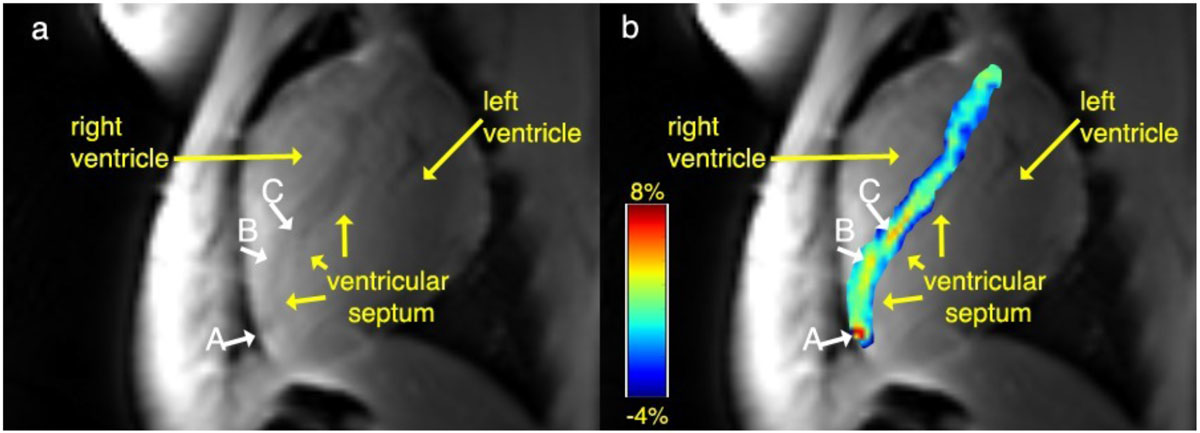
a| An anatomical MR image of the heart showing the right ventricle, left ventricle, and the interventricular septum where the stem cells were transplanted: MSCs expressing HSV1-TK (A) and wild type MSCs (B) were incubated for four hours with 5-MDHT prior to transplantation and control wild type MSCs (C). b| CEST map overlaid on the anatomical image showing labeled MSC^HSV1-TK^ appearing as a hot spot (A).
